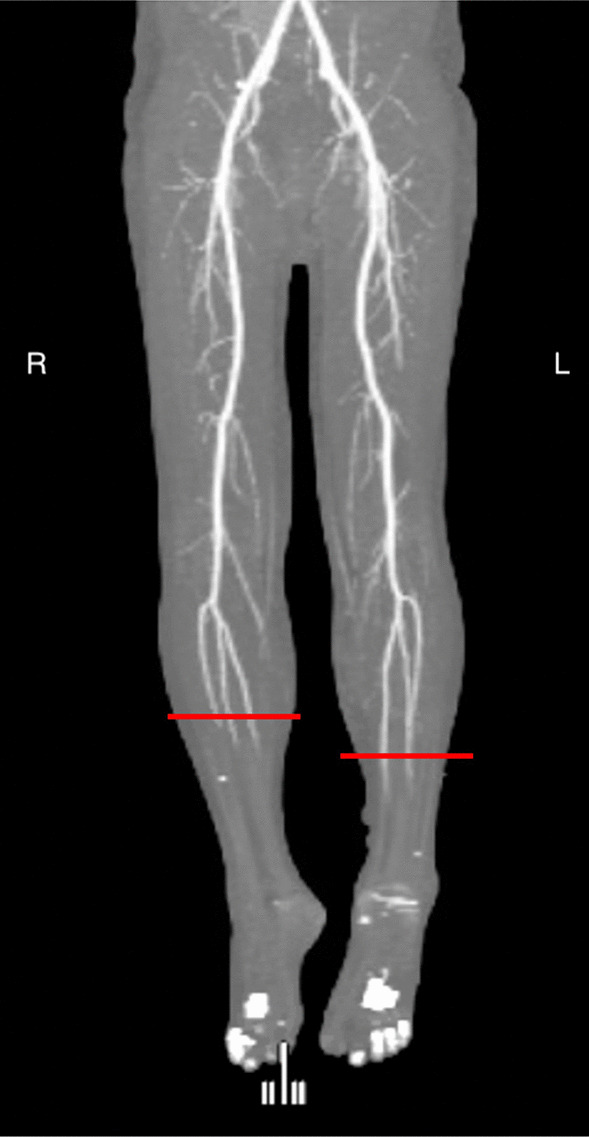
Repeat CT-angiography of the lower limbs (1 week later, during acute admission) demonstrating absent arterial flow past the mid-leg bilaterally. The red lines indicate the point of angiographic “cut-off” of leg blood supply
